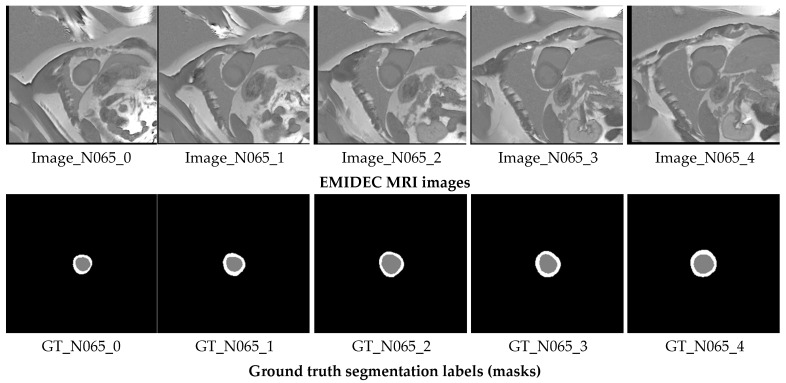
Short-axis MRI slices of a N065 normal patient alongside their corresponding GT segmentation labels. The gray label represents the LV, while the white label corresponds to the Myo.
